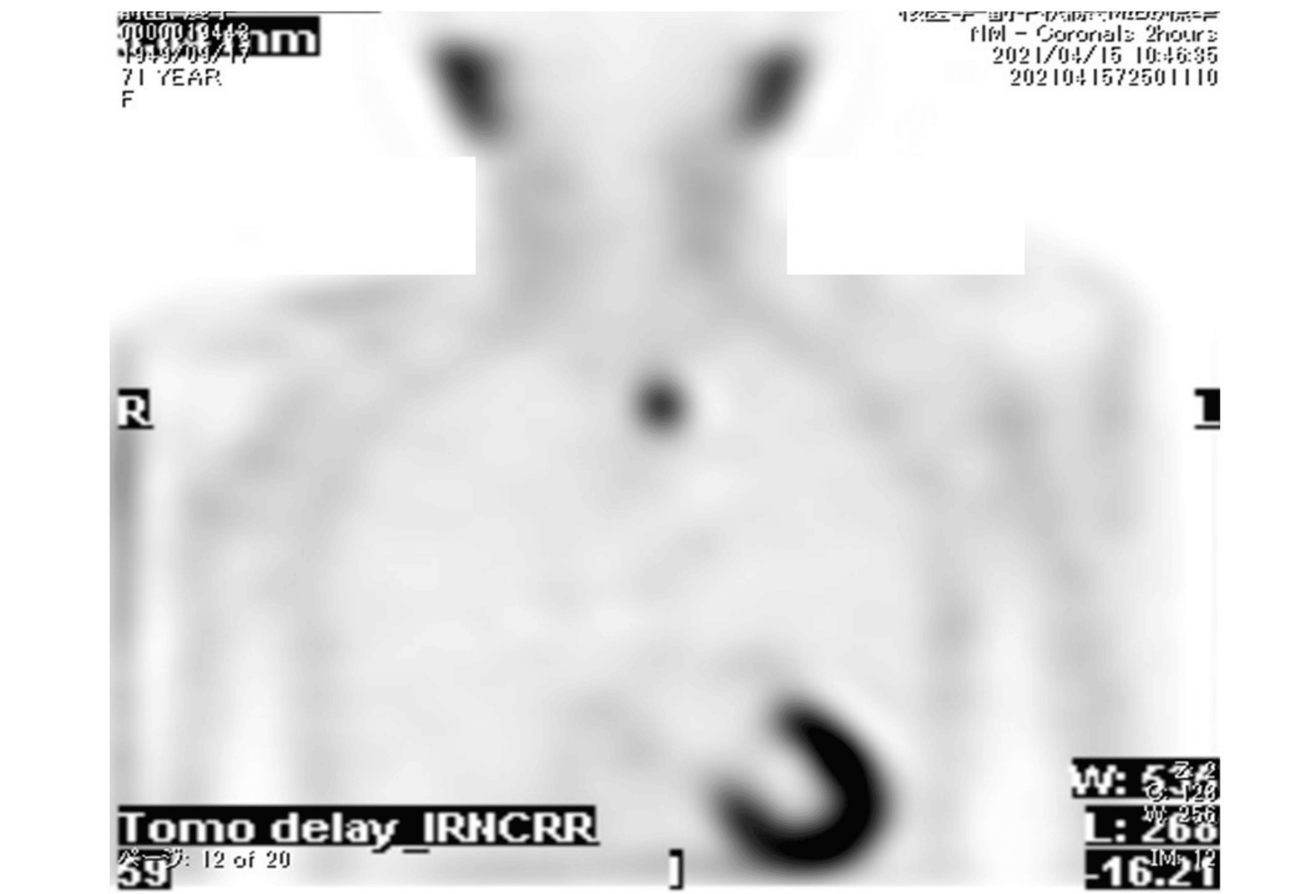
MIBI scintigraphy MIBI scintigraphy reveals a hot spot in the superior mediastinum, located at the caudal end of the left thyroid lobe, suggesting the presence of an ectopic parathyroid adenoma.
